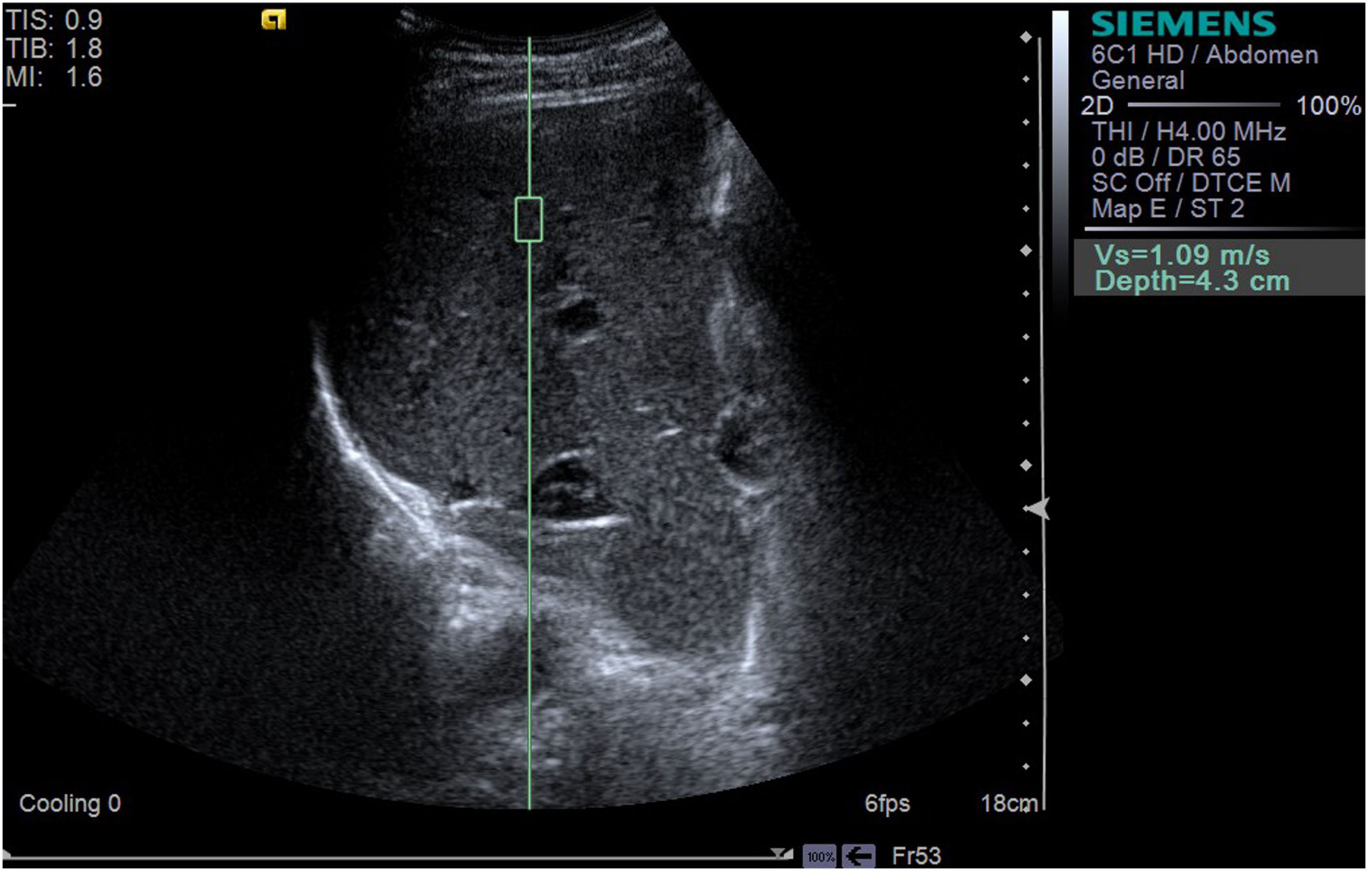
The LSWV of a 61-year-old male shows a LSWV of 1.09 m/s, and ultrasound displays one liver nodule, with a size of 2.8*2.9*3.0 cm^3^. ICG-R15 was 6.0% (<10%); Y-value (multiparametric model) was 4.212 (<16.656).
